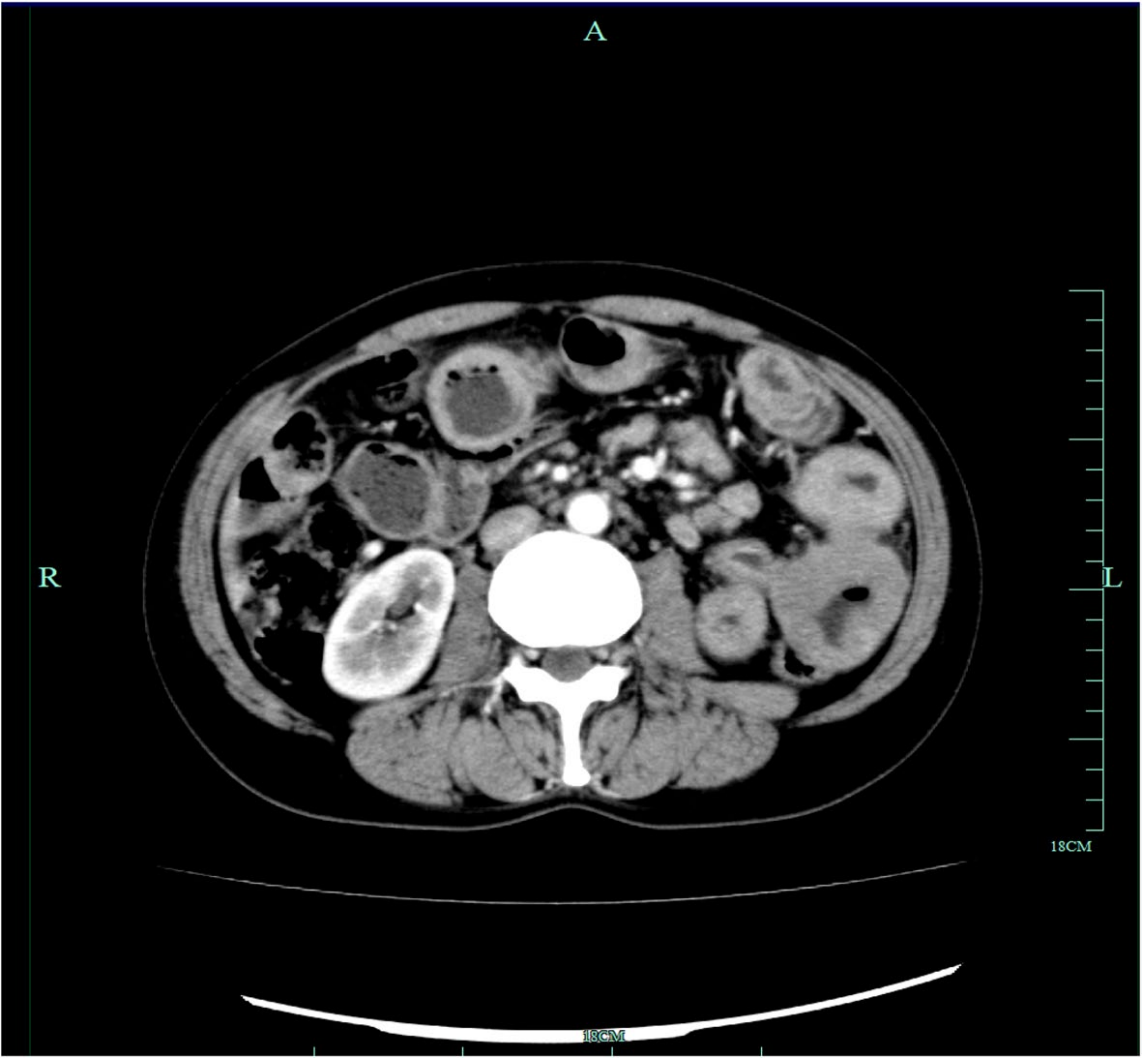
CT enterography showing the aneurysmal dilation
